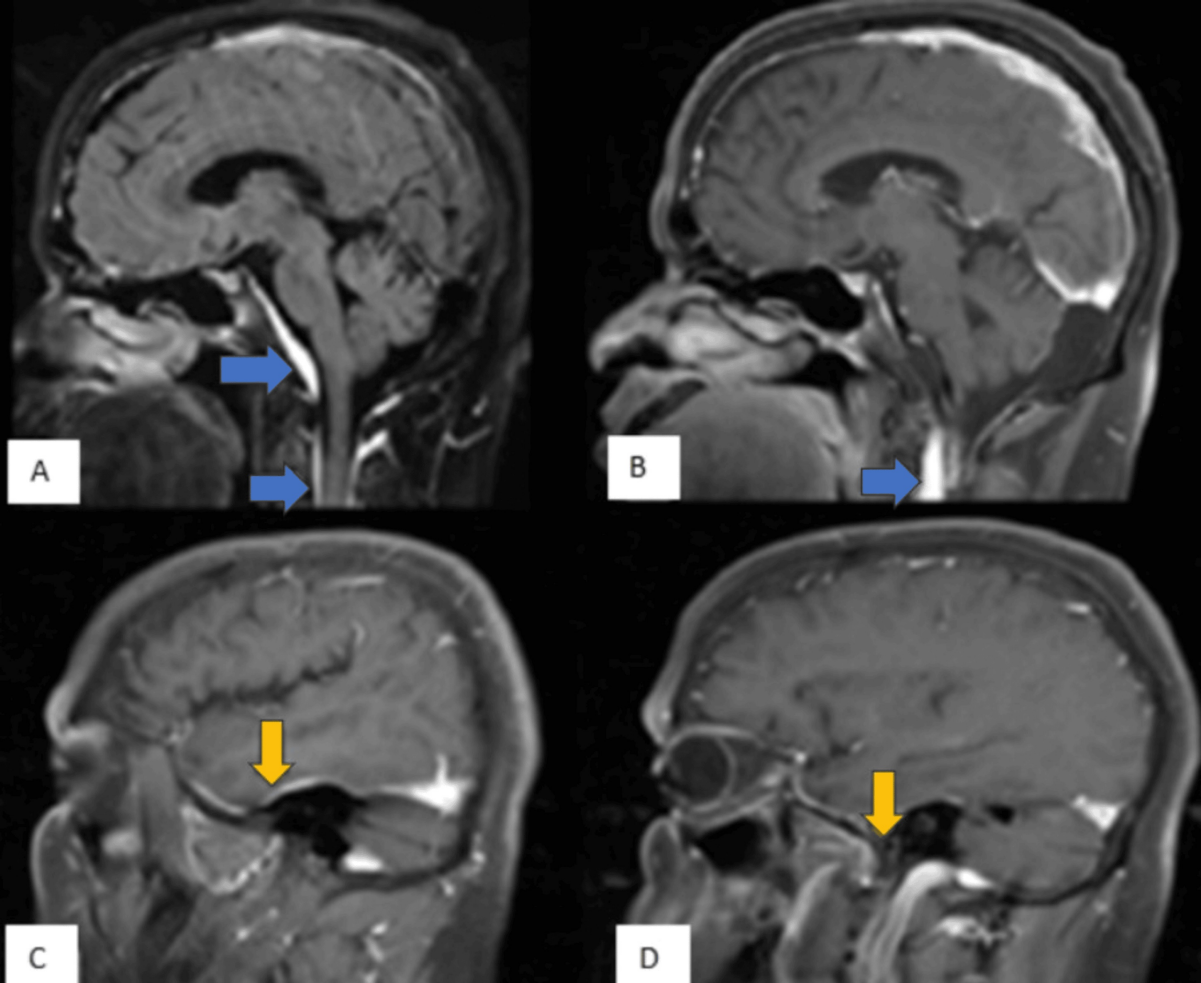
(A) Sagittal FLAIR post-contrast and (B-D) sagittal T1FS post-contrast images show dense pachymeningeal enhancement. Overlying the clivus and in the upper spinal canal, just anterior and posterior to the upper cervical cord, are marked by blue arrows (A, B). Yellow arrows (C, D) indicate the location of both temporal lobes. FLAIR: Fluid-attenuated inversion recovery; T1FS: T1-weighted fat saturated.
